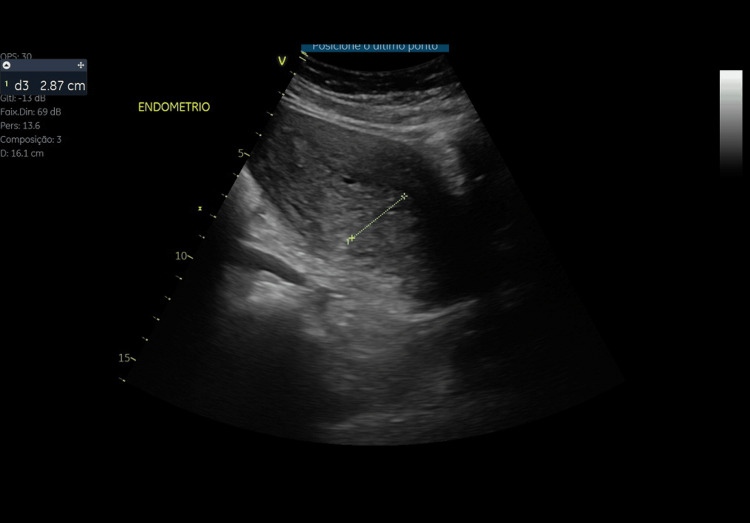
Transabdominal and transvaginal pelvic ultrasound demonstrating heterogeneous material within the endometrial cavity, consistent with retained products of conception.
